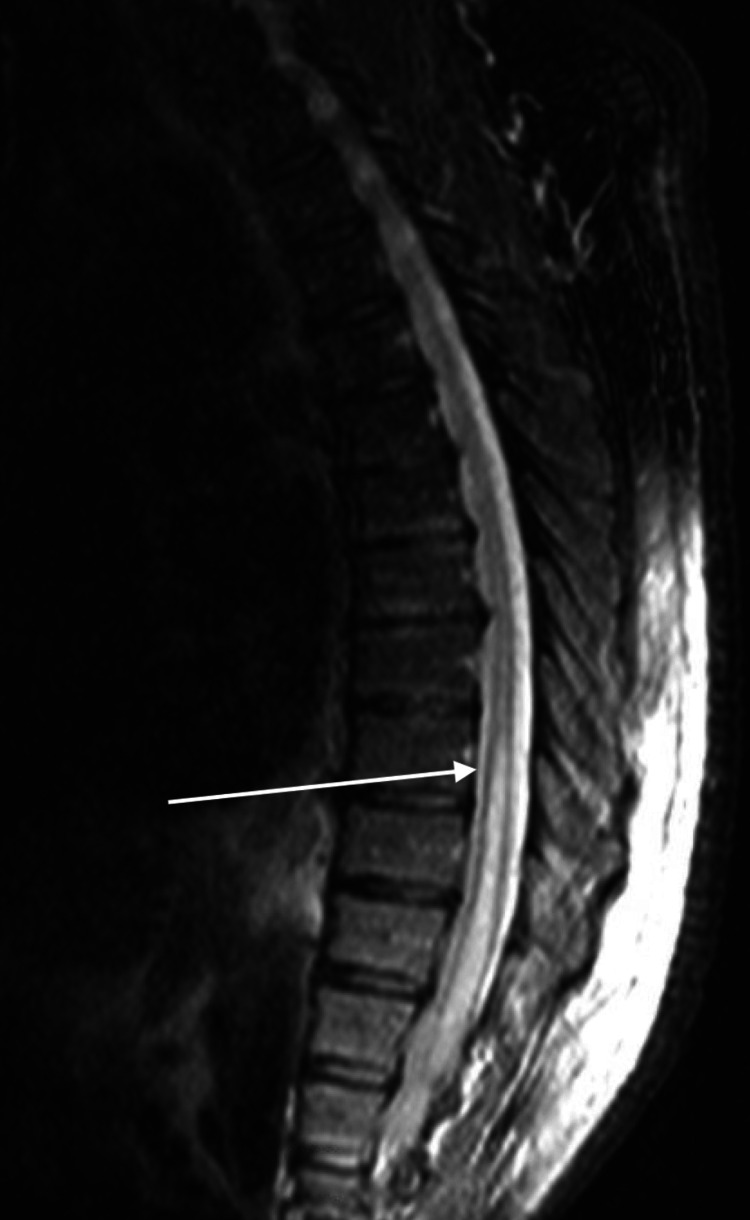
Repeat magnetic resonance imaging of the thoracic spine with fat suppression showing hyperintensity from T5 to conus
